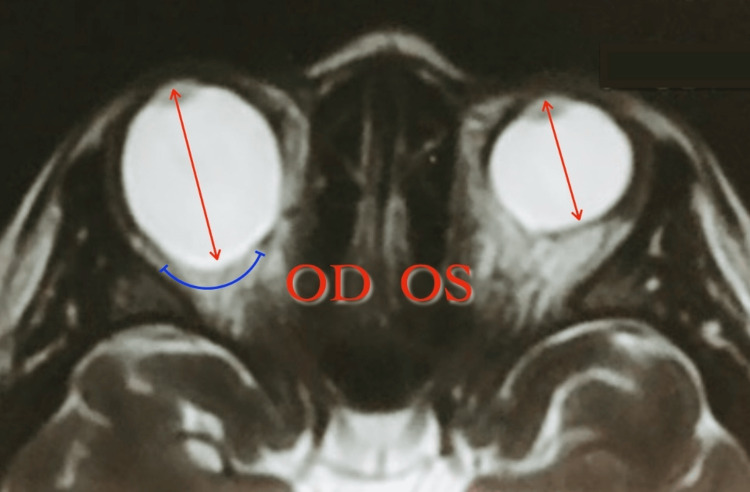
T2-weighted MRI T2-weighted MRI shows a difference in axial length (red arrows) and subtle curvature of posterior staphyloma in the right eye (blue line). Axial length: 34 mm OD; 24.04 mm OS OD: Right eye; OS: Left eye
